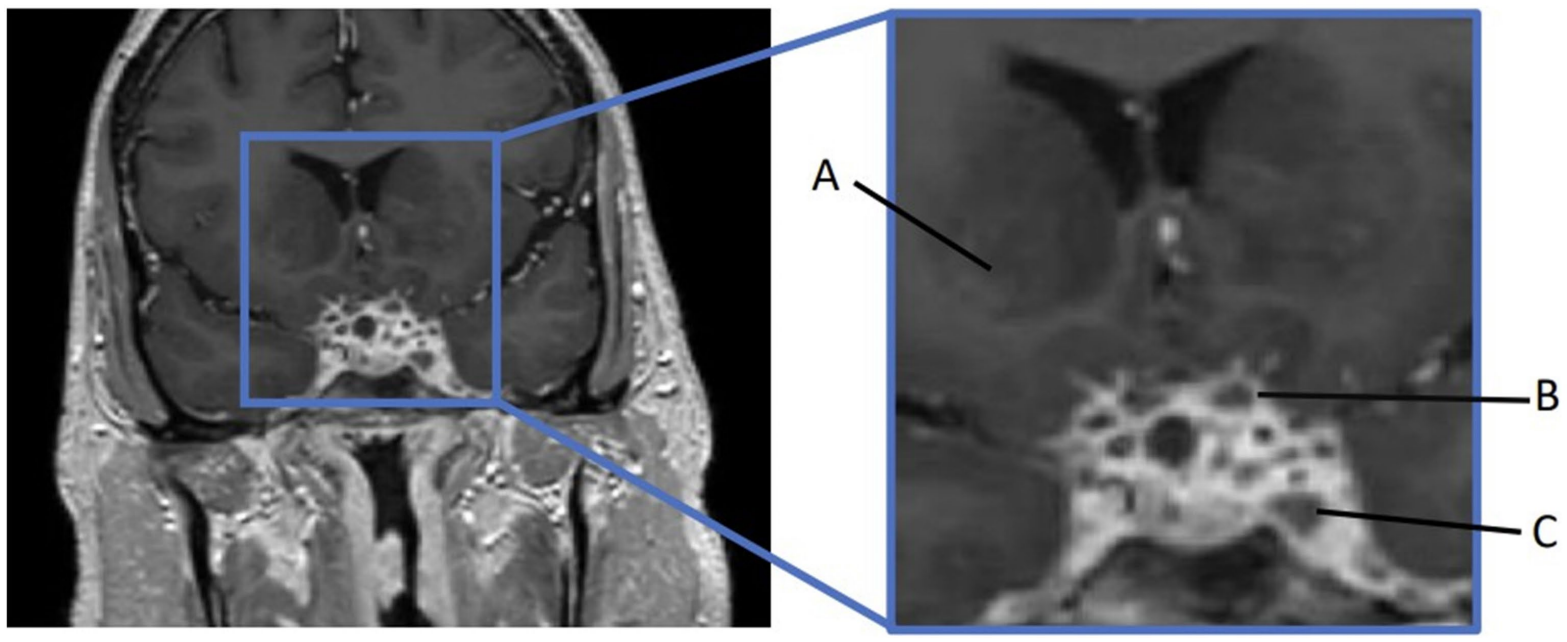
MRI skull base T1 + C at 6-week follow-up with reported improvement of symptoms. (A) Interval increase in the cavernous sinus mass size with extension into the pituitary fossa. (B) Subtle enhancement along V2 on the left, suggesting infiltrative process(inlet). (C) Narrowing of the left ICA.
